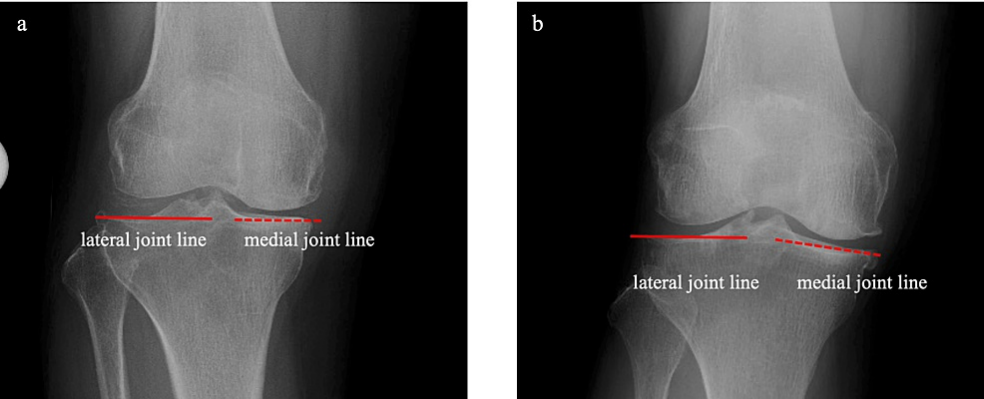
Preoperative radiographic classification (a) Leveled type: The medial and lateral tibial surfaces are aligned. In this type, the medial tibial surface represents the CJL obliquity. (b) Uneven type: The two tibial surfaces are not aligned. The posterior condylar axis is used to define the CJL obliquity. CJL: Coronal joint line.
